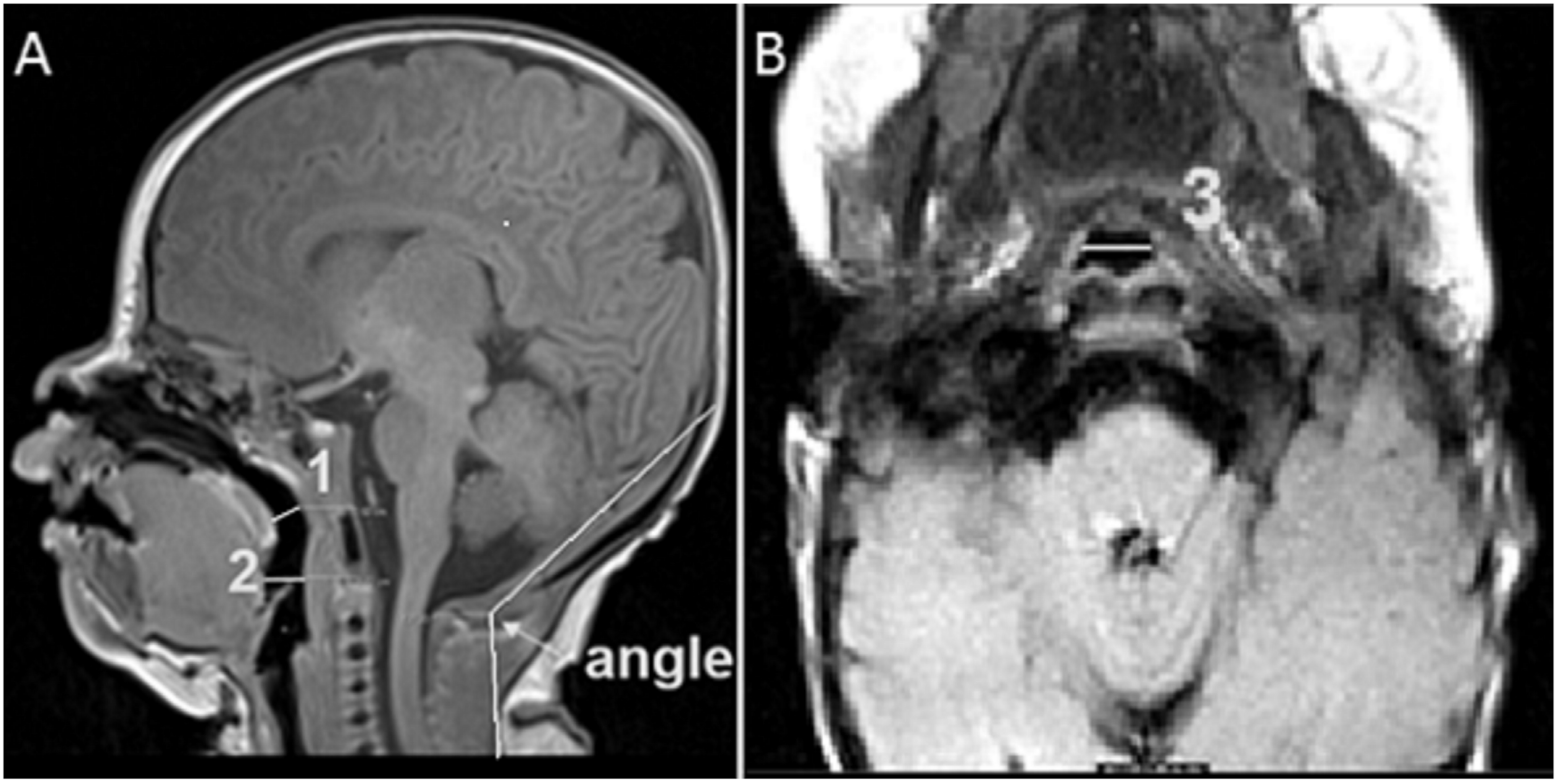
A—T1-weighted MR image of head-neck region of a newborn infant showing head-tilt angle between the occipito-ophisthion-C7 spinous process, AP diameters of airway at the level of palate (1) and tongue (2) in mid-sagittal plane. B—Axial T1-weighted MR image of head-neck region of a newborn infant showing lateral diameter of airway at the tongue level (3).
